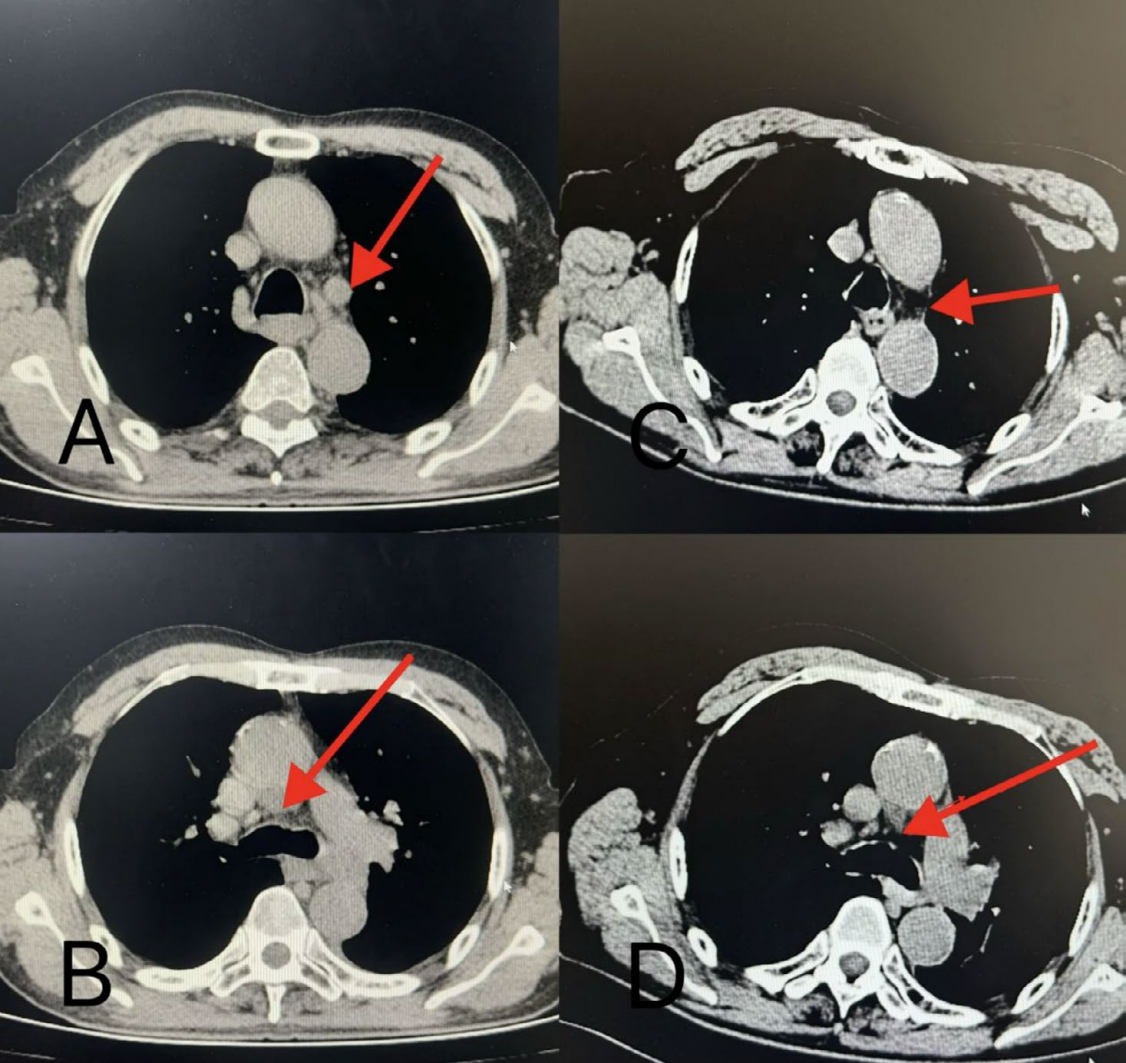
Changes of lymph nodes under CT. The lung CT scan showed that mediastinal lymph node and hilar metastasis were significantly smaller than before. 2023/6/22 Chest CT: Enlarged lymph nodes were seen beside the left hilum of the lung (A); 2023/6/22 Chest CT: Enlarged lymph nodes could be seen in the mediastinum (B); 2024/4/21 Chest CT: No significantly enlarged lymph nodes were observed beside the left hilum of the lung (C); 2024/4/21 Chest CT: No significantly enlarged lymph nodes were found in the mediastinum (D).
